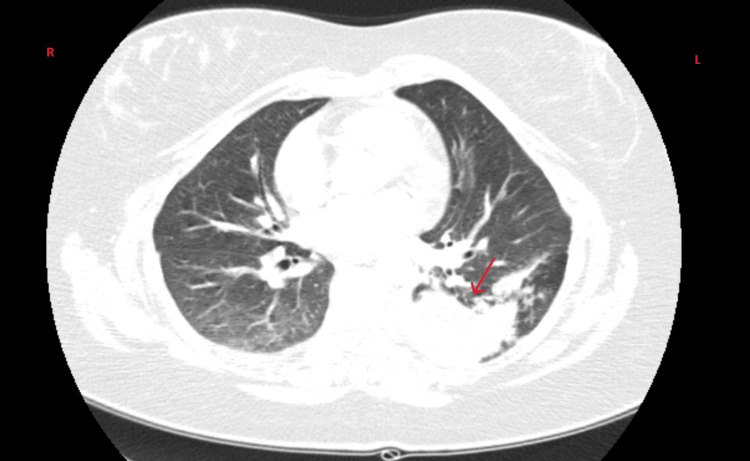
Computed tomography of the chest at emergency department presentation, showing ground-glass opacification and consolidation of the left lower lobe (red arrow), consistent with an acute inflammatory/infectious process (pneumonia). The underlying bronchial atresia is not readily apparent due to active inflammation.
